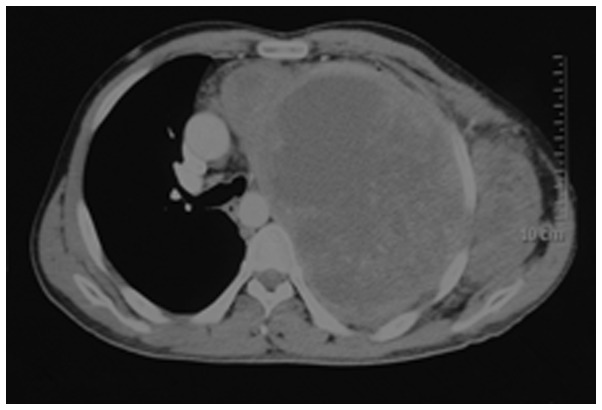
Axial computed tomography (CT) image showing a large mass infiltrating the chest wall with 2nd rib destruction.
